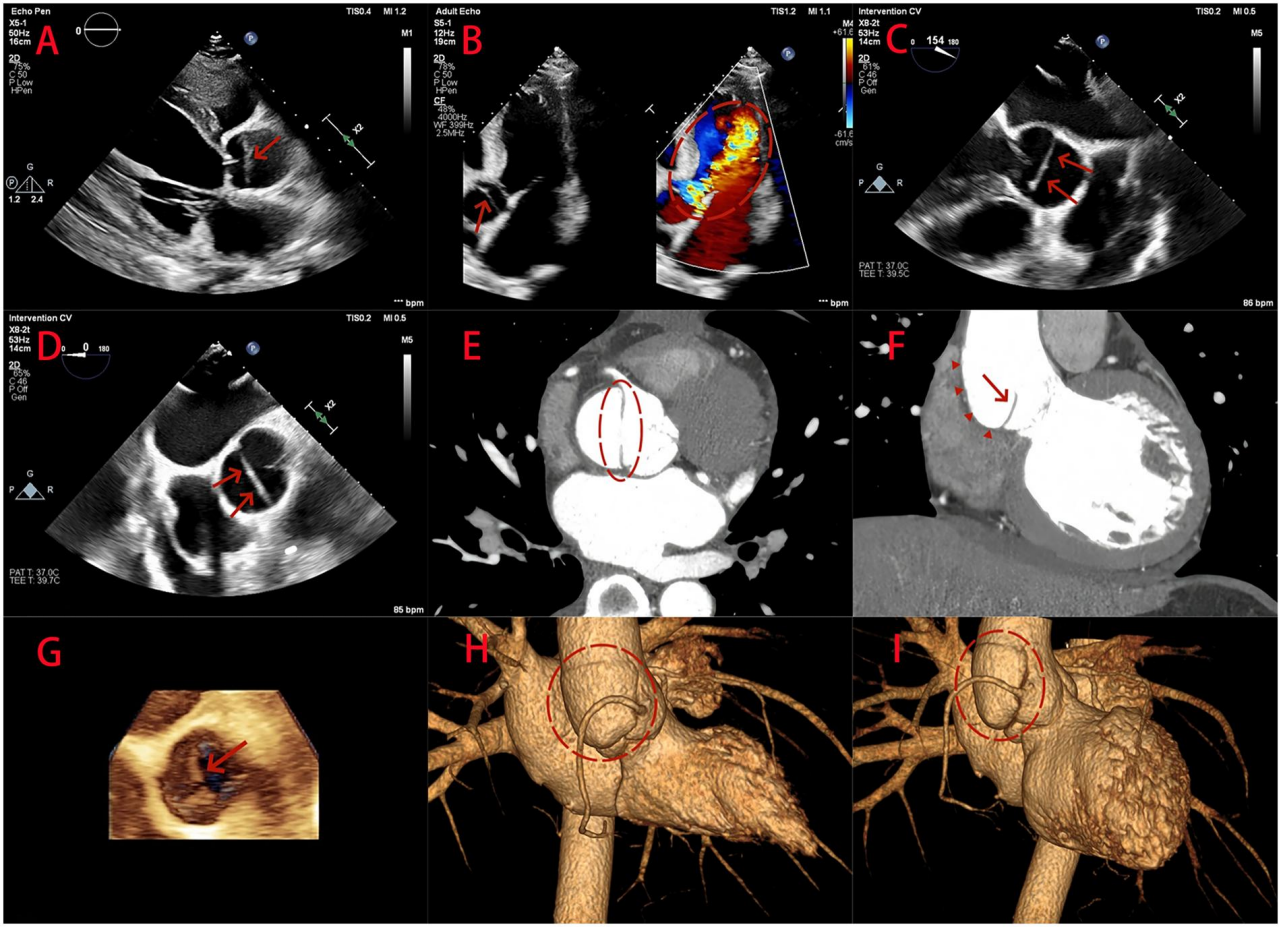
Echocardiographic and coronary CTA findings in a 36-year-old man with localized aortic root dissection. (A) Admission transthoracic echocardiography (TTE) shows a membrane-like structure (red arrow) at the sinotubular junction of a non-dilated aortic root, oriented perpendicular to the long axis of the ascending aorta. (B) Apical five-chamber view demonstrates the membrane-like structure (red arrow) more clearly, with significant regurgitation originating from both the membrane and the aortic valve (red circle). (C,D,G) Preoperative transesophageal echocardiography (TEE) in two- and three-dimensional views delineates the membrane-like structure (red arrow), extending toward the left–noncoronary commissure and into the right coronary sinus. (E,F) Retrospective review of the preoperative coronary CTA shows a localized, obliquely oriented intimal flap at the aortic root (red arrow) on coronal and axial images, associated with focal outward bulging of the aortic wall (red triangle). (H,I) Three-dimensional reconstructions demonstrate focal outward bulging of the adjacent aortic root wall in the dissected segment (red circle).
